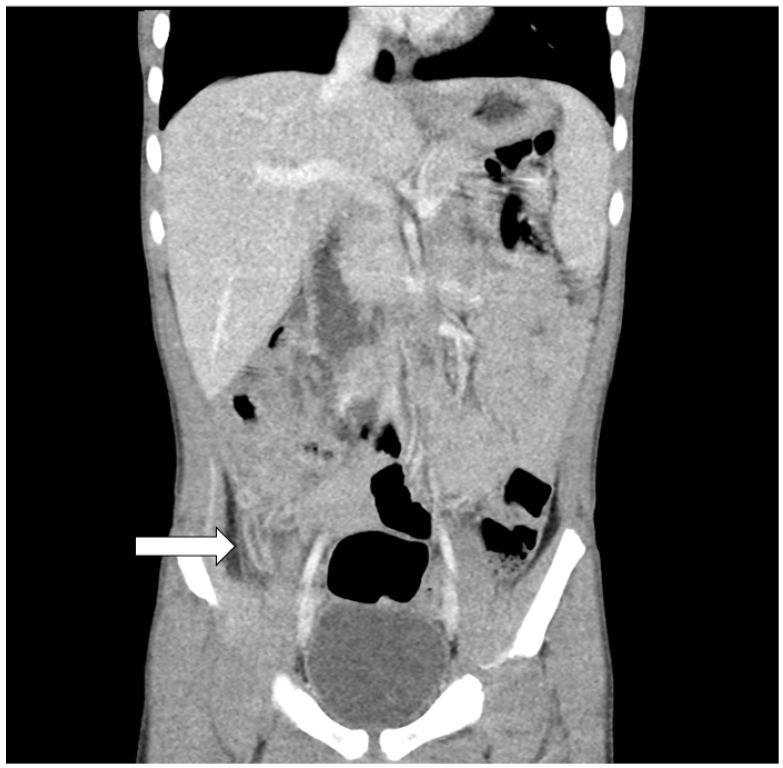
Computed tomography of abdomen demonstrated appendicitis (arrow).
